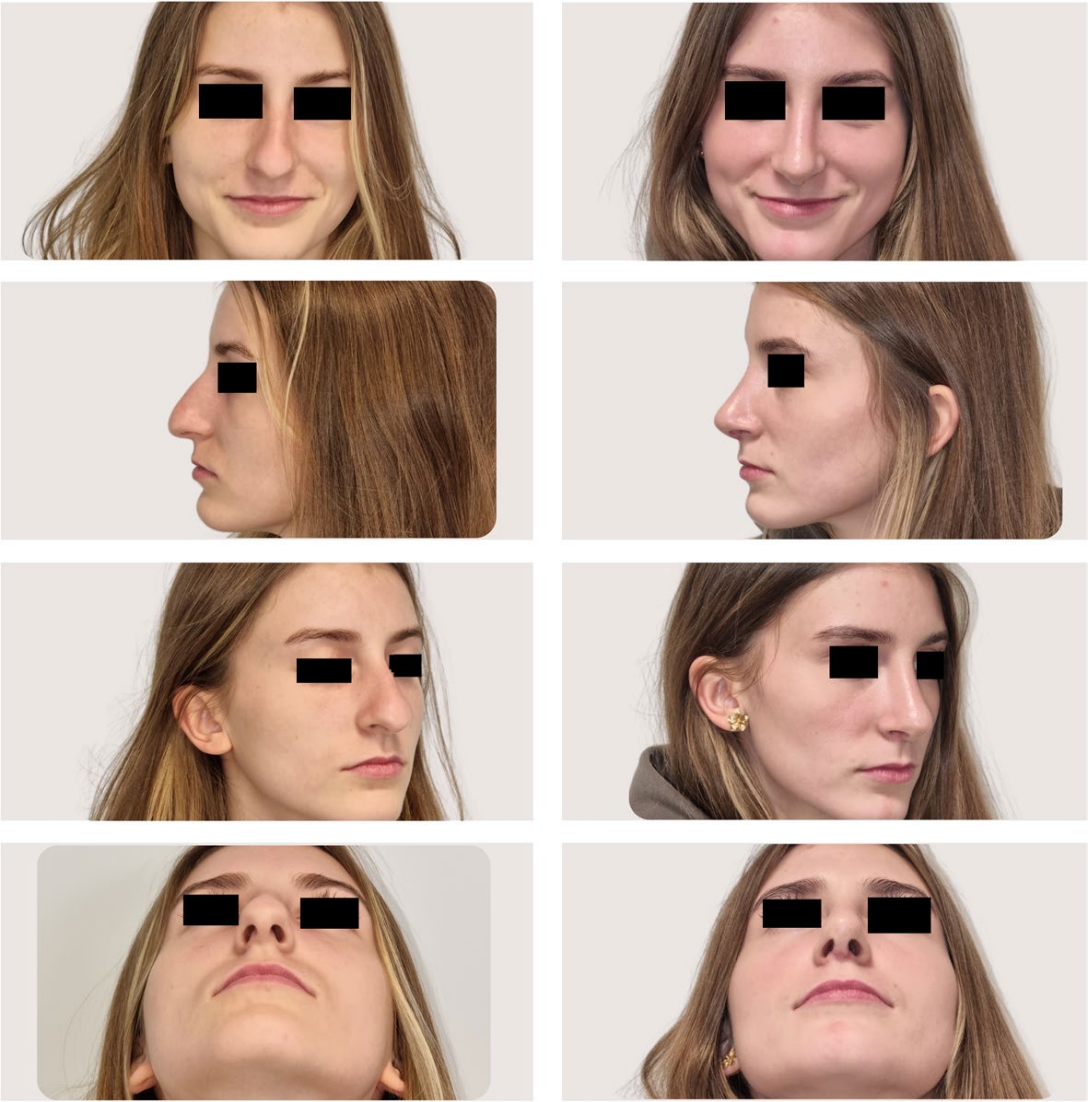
CT-assisted precision rhinoplasty in patient A desiring natural feminine harmonious aesthetic outcome
